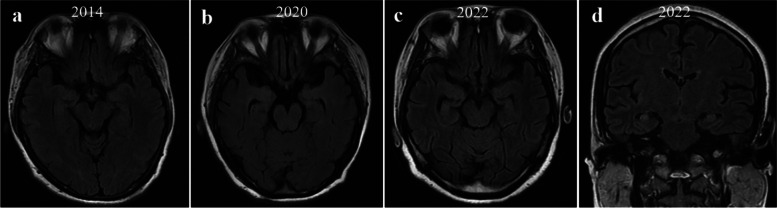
Brain MRI images of the patient in 2014, 2020, and 2022. a, b Brain MRI was normal, and bilateral hippocampal volume was normal in the cross-sectional images in 2014 (a) and 2020 (b). (c) Bilateral hippocampal volume was decreased in the cross-sectional images in 2022. d Brain MRI showed suspicious hyperintensity in the bilateral hippocampi in the coronal image in 2022
